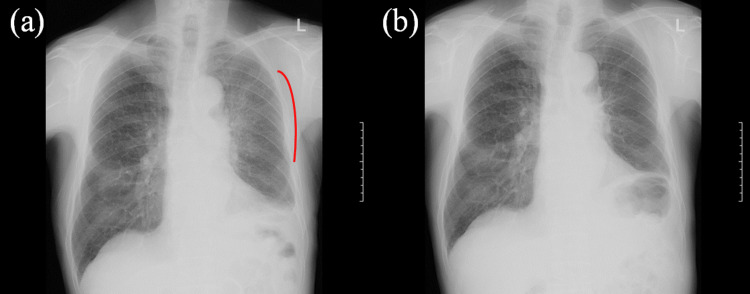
X-ray photographs X-ray photograph (a) with a clear consolidation shadow marked with a red line in the left upper lung field, which was acquired on the day the CBCT images suggested RP. Then, oral prednisolone administration was started. Another X-ray photograph (b) was acquired a week later, the shadow being undetected. CBCT - cone-beam computed tomography; RP - radiation pneumonitis
